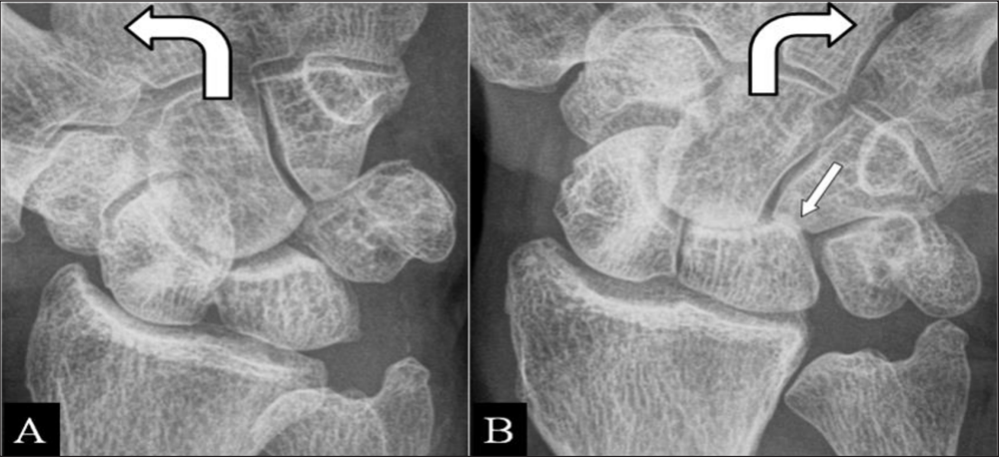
Hamatolunar abutment. (A) PA plain mobility radiographs in radial and ulnar deviation. (A, B) Step-off at the lunotriquetral joint of the first and second line of Gilula. (B) Secondary impaction of the hamate and lunate bone in ulnar deviation (oblique arrow).
